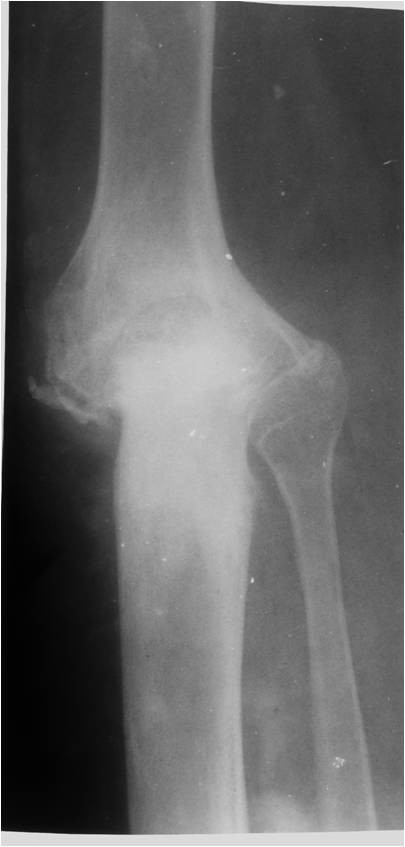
AP radiograph showing bony union at the docking site.
